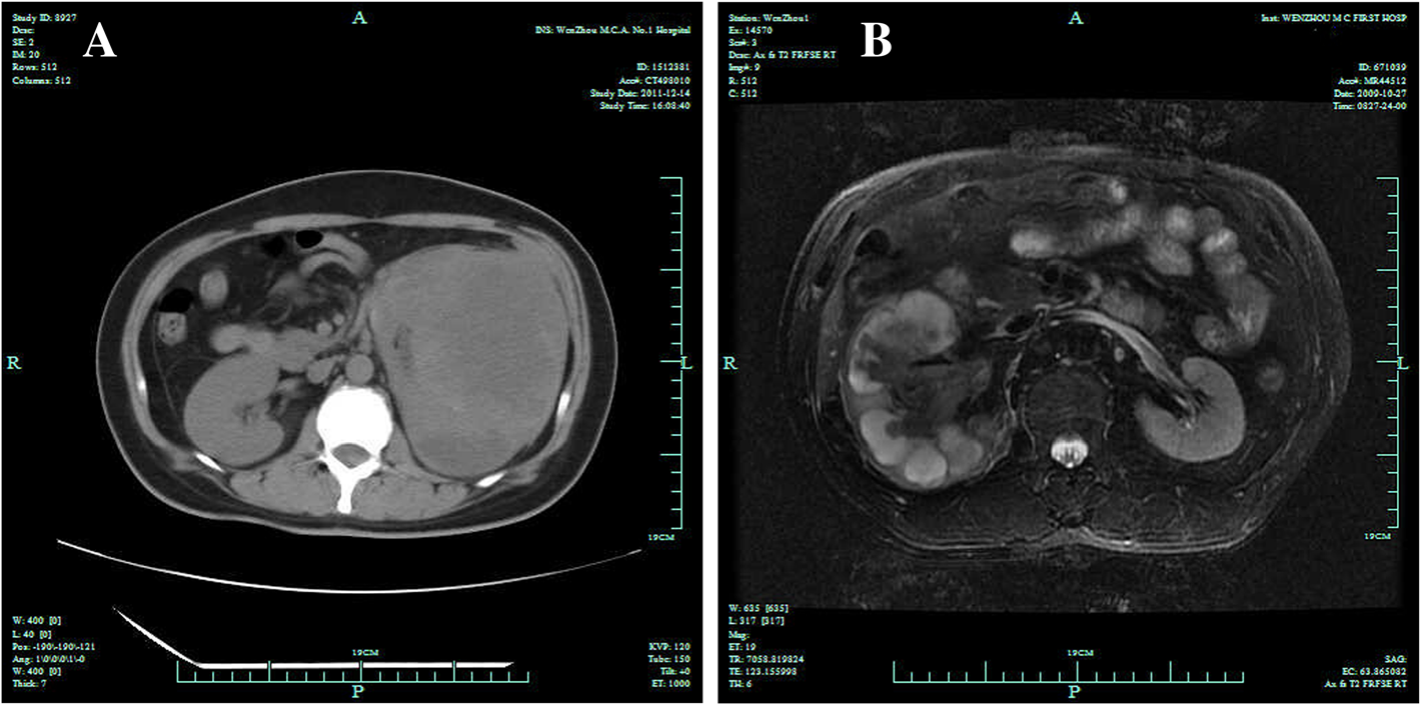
Scans of the left kidney mass. A. CT scan: a mass measuring 8 × 10 cm in the left kidney. B. MR: A giant abnorminal signal in the right kidney, measuring 9 × 10 cm. Hydronephrosis existed.
